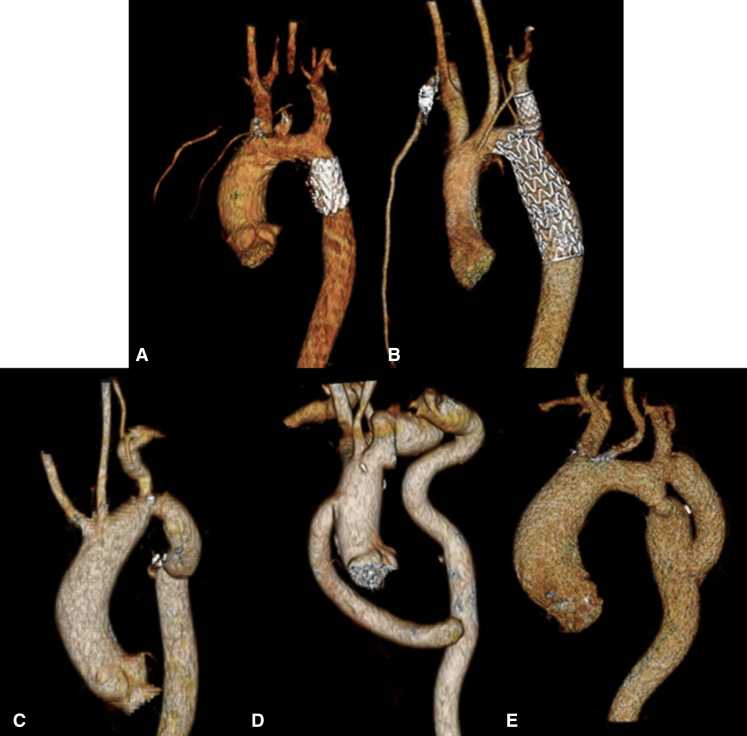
Postoperative computed tomography of patients with primary coarctation repaired via balloon-expandable stent (A), thoracic branched endoprosthesis (B), interposition graft (C), ascending–descending extra-anatomic bypass (D), and arch–descending extra-anatomic bypass (E).
